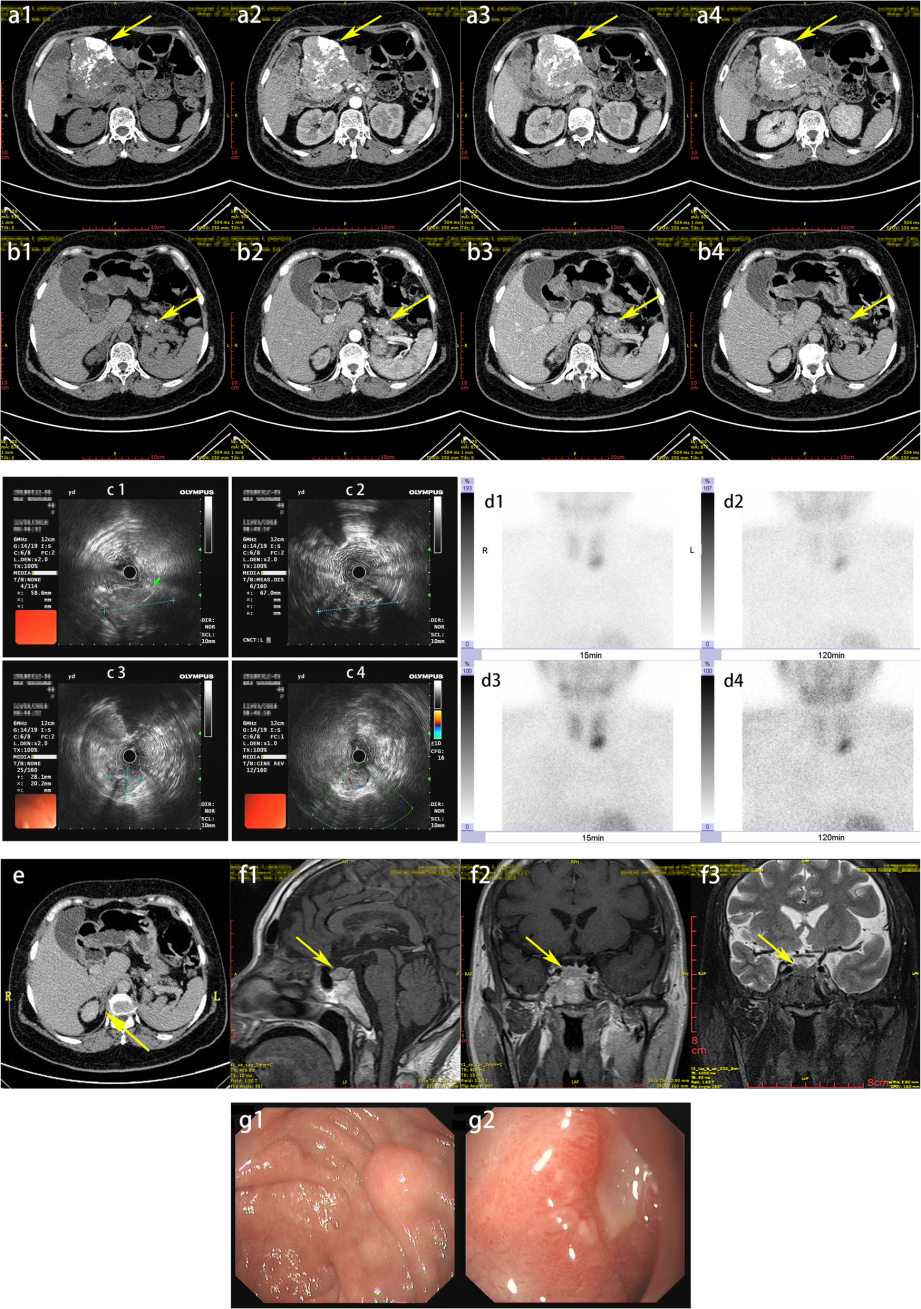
The proband’s clinical imageology examination images. A a1–a4: Pancreatic neck lesions (8.0 × 6.8 × 8.4 cm) (a1, plain scan; a2, arterial phase; a3, portal phase; a4, delayed phase). B b1–b4: Ring enhanced nodule at the tail of the pancreas (2.8 × 2.7 cm) (b1, plain scan; b2, arterial phase; b3, portal phase; b4, delayed phase). C The hypoechoic mass and the internal echoes were mixed. c1, c2: head of the pancreas (cannot be observed in the full field); c3, c4: the tail of the pancreas (2.81 × 2.02 cm). D d1, d3: 15 min after the 99mTc-stabimidine (MIBI) injection, an area of increased imaging agent appeared in the lower lobe of the left thyroid; d2, d4: 2 h after the injection, the area of distribution of the imaging agent under the left lobe of the thyroid did not decrease significantly. E Abdominal CT result showing that a mass occupied the right adrenal gland. F f1–f3. Pituitary-enhanced MRI examination showing that the hypophysis was full in shape (height 0.95 cm). f1, f2: T1WI; f3: T2WI. f1: sagittal view; f2, f3: coronal view. G g1, Multiple submucosal uplifts (the larger was about 0.6 × 0.8 cm) at the junction of the duodenal bulb and the descending part of the duodenum; g2, the distal shallow ulcer in the descending part of the duodenum was about 1.0 × 0.5 cm in size
